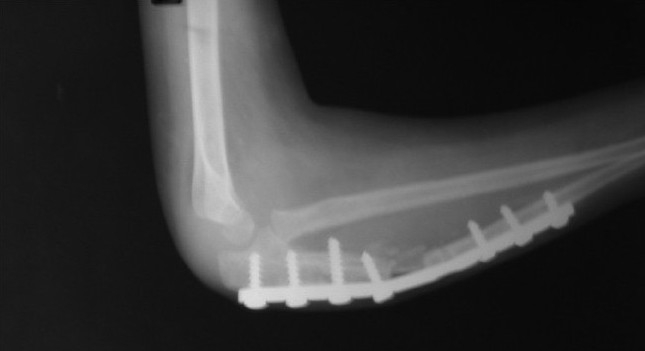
Lateral radiograph of elbow and forearm after open reduction, ulna osteotomy, bone grafing and annular ligament reconstruction
